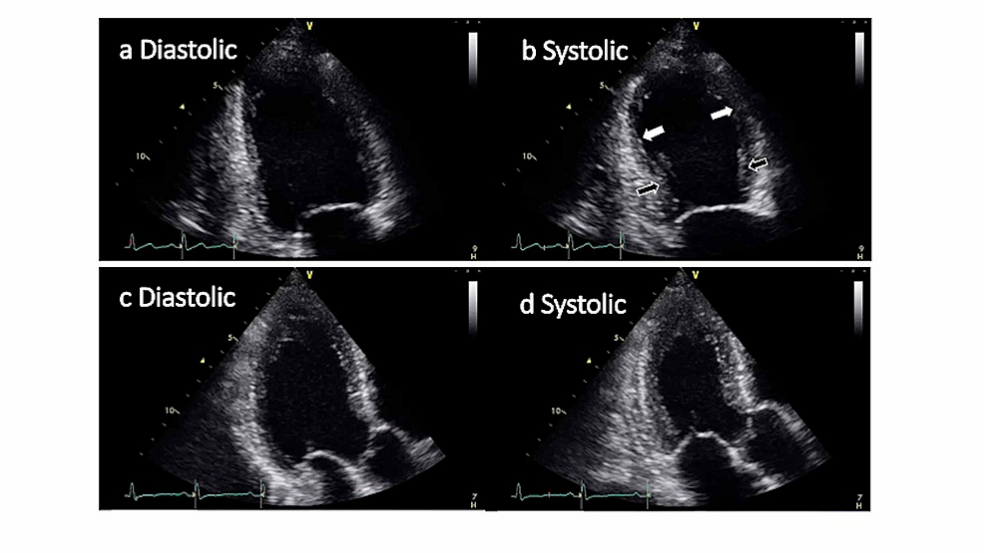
Transthoracic echocardiography a-b: Transthoracic echocardiography (TTE) on admission showed left ventricular desynchrony with decreased ejection fraction. The base of the left ventricle contracture was hyperkinetic (black arrowhead), while the rest of the left ventricle was akinetic or dyskinetic (white arrowhead). c-d: TTE on the third hospital day revealed that left ventricular dysfunction had recovered completely.
